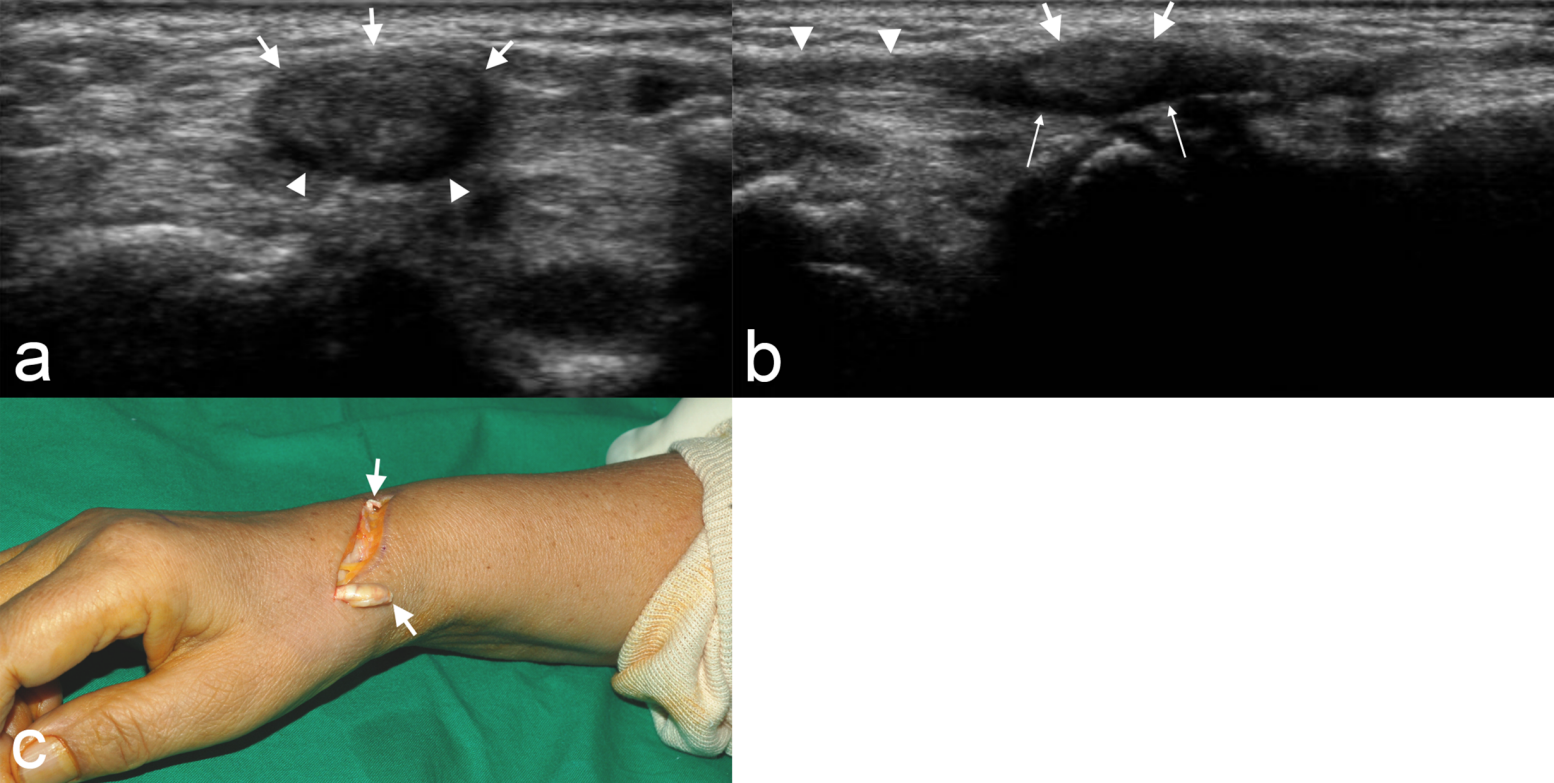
A 63-year-old woman with a rupture of the extensor pollicis longus tendon. (a) Ultrasound image of transverse scan shows pseudomass formation (arrows) that reveals an ovoid-shaped, heterogeneously hypoechoic lesion with a peripheral anechoic portion indicating fluid collection (arrowheads). (b) On ultrasound image of longitudinal scan, the extensor pollicis longus tendon shows decreased echogenicity at the ruptured tendon site (arrowheads). The ruptured tendon stump is seen as pseudomass formation (arrows) with surrounding anechoic portion indicating fluid collection (thin long arrows). (c) On surgery, the rupture of the extensor pollicis longus tendon was confirmed (arrows). Pseudomass formation was occupied with granulation tissue and hemorrhage intraoperatively.
